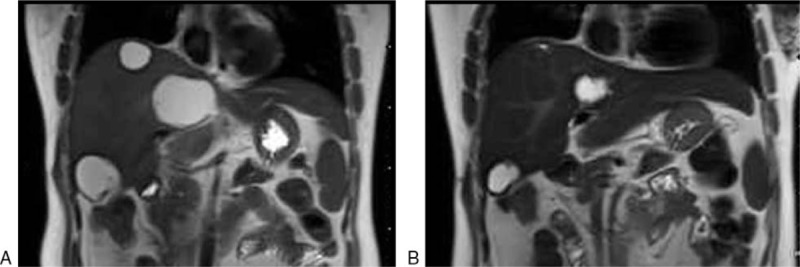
Changes of liver metastases before (A) and after (B) transcatheter hepatic artery chemoembolization treatment by abdominal magnetic resonance imaging.
